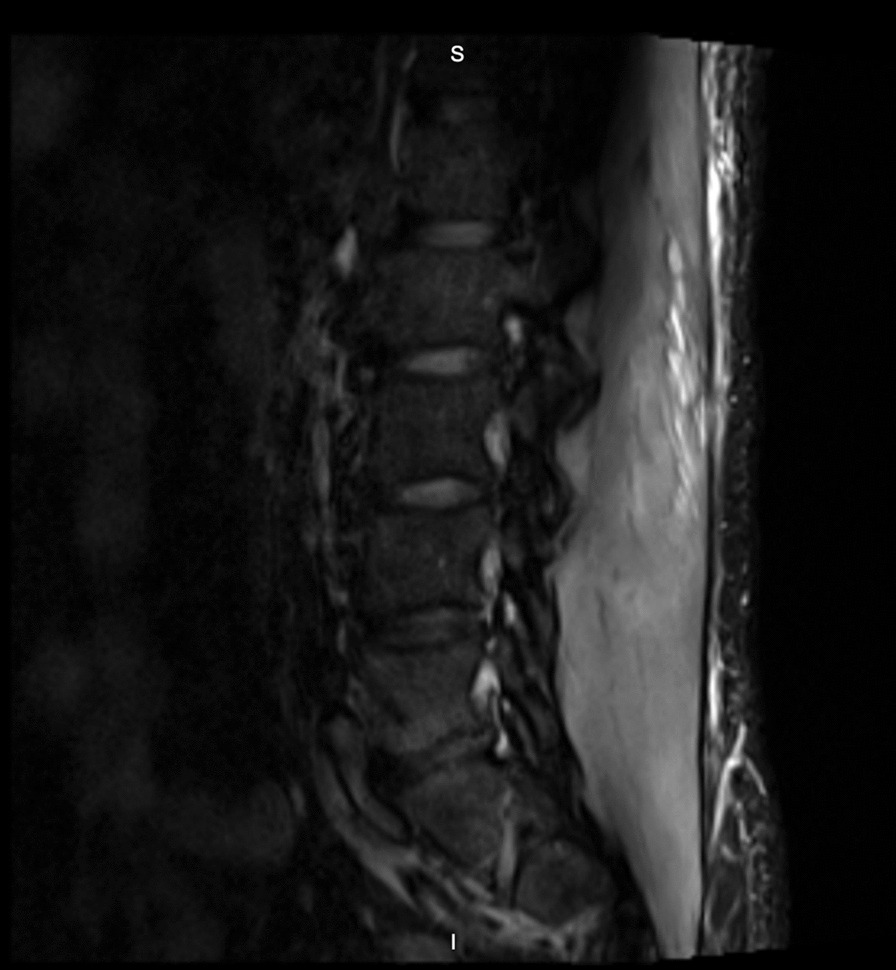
Sagittal STIR MR image of the lumbar spine demonstrating profound paralumbar edema
